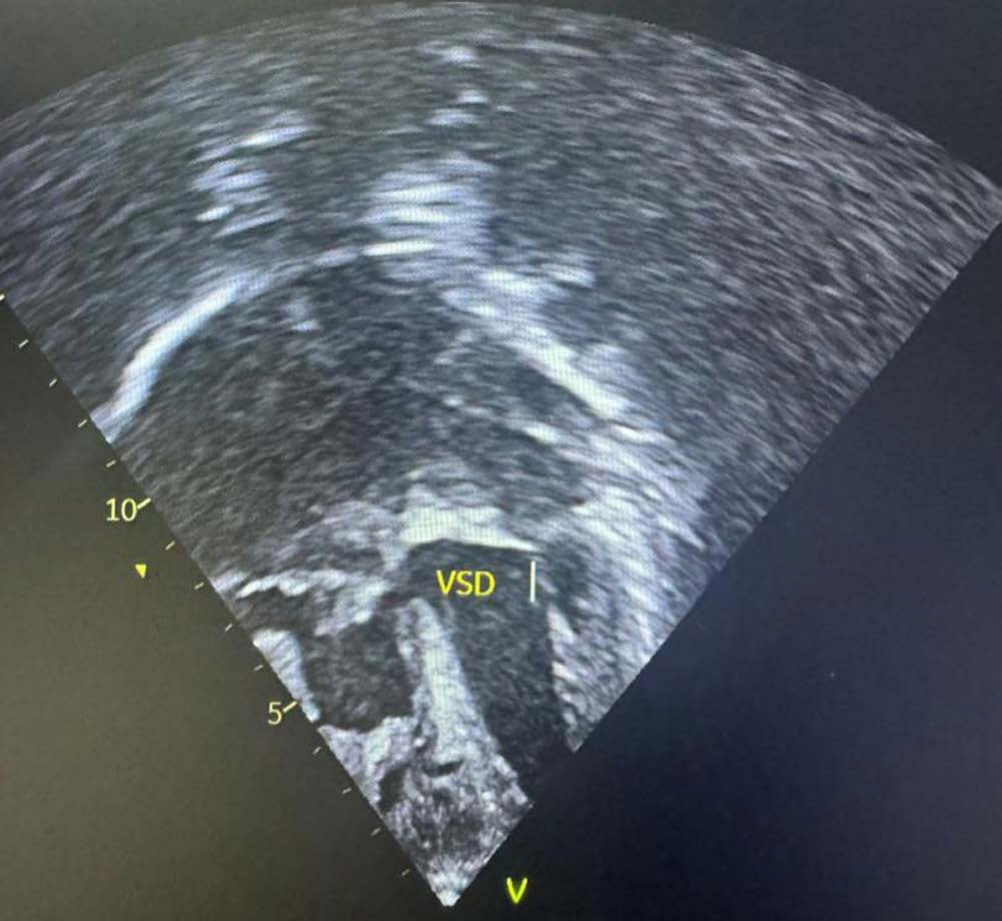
Echocardiographic image showing ventricular septal defect (VSD).
